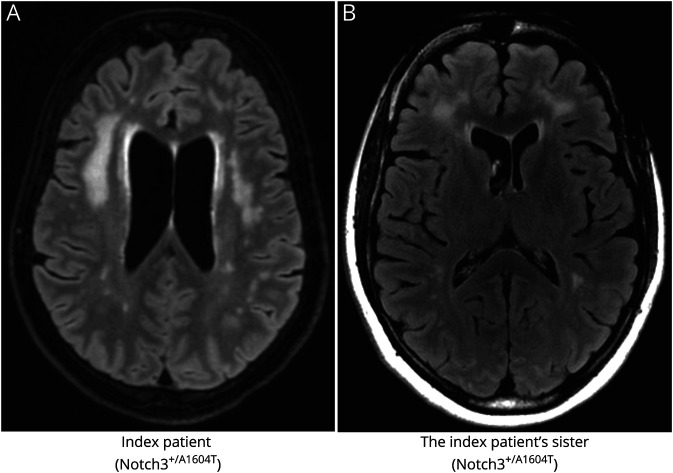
MRIs From 2 Siblings Heterozygous for the NOTCH3^A1604T^ Mutation The index patient (A) was subjected to axial 3D fluid-attenuated inversion recovery (FLAIR). Confluent WMLs were found predominantly in the frontal subcortical and deep white matter. No lesions where found in the anterior temporal region and only a few small lesions in capsula externa. The index patient's heterozygous sister (B) was subjected to axial 2D FLAIR. A similar lesion localization as in the index patient was observed but with somewhat smaller lesion load. Bifrontal predilection, with no lesions in the anterior temporal area, and few lesions in capsula externa were also observed. WML = white matter lesion.
